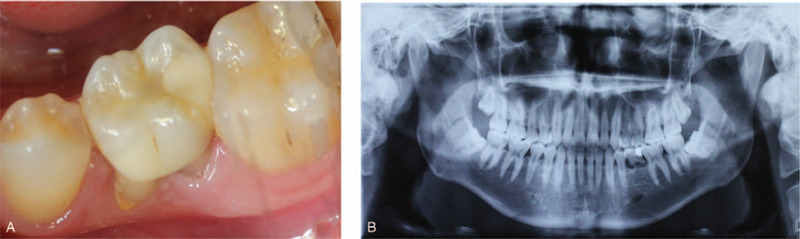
Preoperative and intraoperative photographs of case 2. (A) Clinical view of the tooth 75; (B) radiographic aspect of the tooth.
